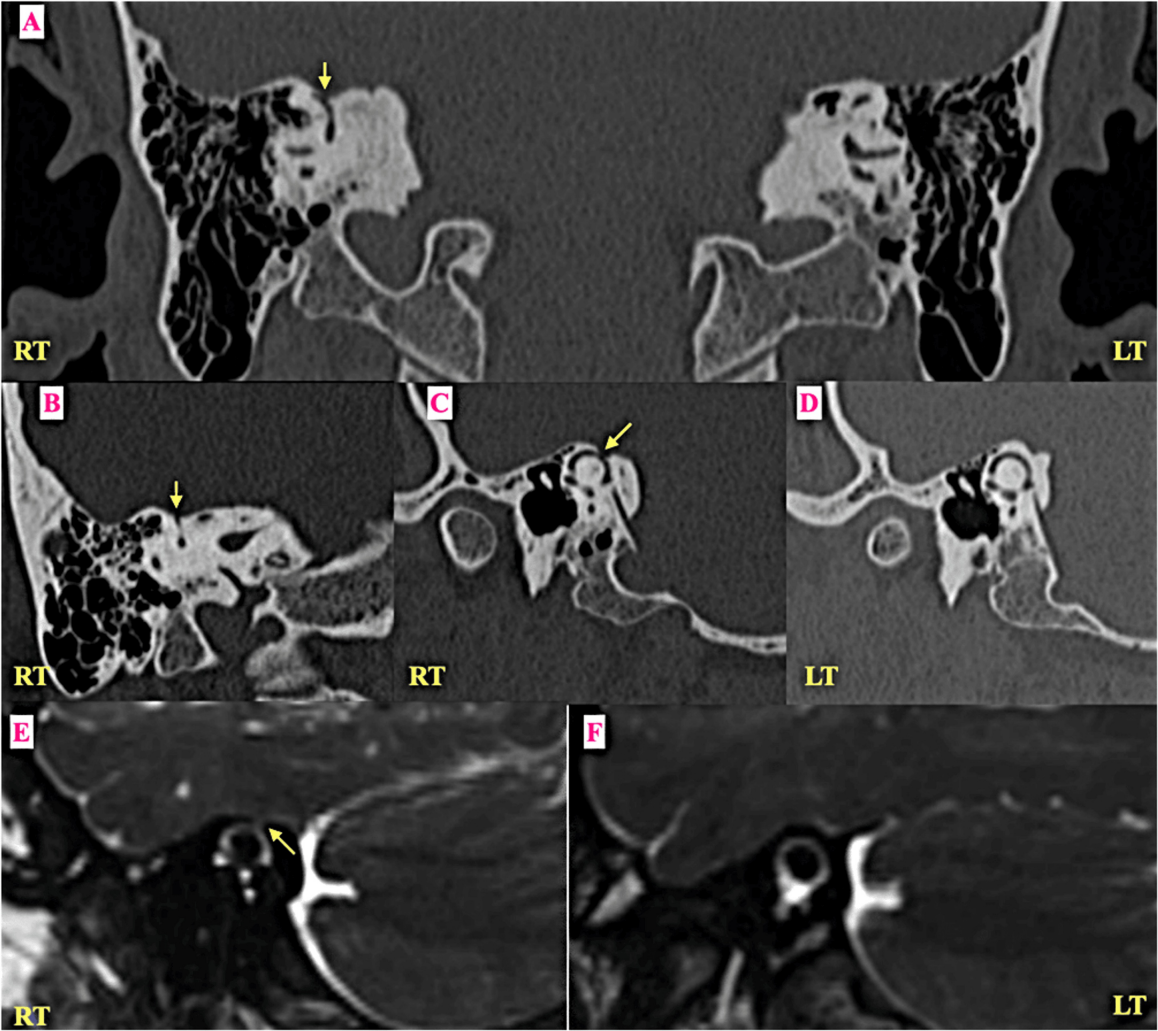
Right-side unilateral dehiscence of superior semicircular canal (A) Coronal high-resolution CT (HRCT) image of the temporal bone displaying a small area of bony dehiscence (yellow arrow) over the superior semicircular canal on the right side. A normal thick bony rim is seen overlying the left superior semicircular canal. (B) HRCT image of the right temporal bone in “Stenver’s plane” showing the bony dehiscence (yellow arrow) over the right superior semicircular canal. (C) HRCT image of the right temporal bone in “Poschl’s plane” showing a small area of bony dehiscence (yellow arrow) over the right superior semicircular canal. (D) The HRCT image of the left temporal bone in “Poschl’s plane” showing a normal thick bony cover over the left superior semicircular canal. (E) 2D reformation of a 3D Constructive Interference in Steady State (CISS) image of the right temporal bone in “Poschl’s plane” showing a small area of bony dehiscence (yellow arrow) over the right superior semicircular canal. (F) 2D reformation of a 3D CISS image of the left temporal bone in “Poschl’s plane” showing a normal bony covering over the left superior semicircular canal.
